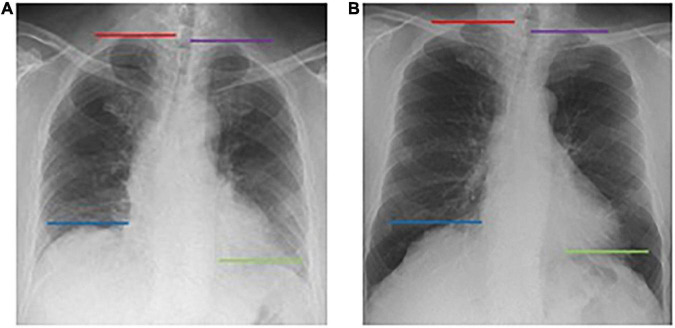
The Kinetic Analysis Tool™ automatically recognizes the bilateral pulmonary apexes and diaphragm dome. Dynamic X-ray working station automatically marked the bilateral diaphragm dome (right diaphragm: blue line; left diaphragm: green line) and lung apex (right lung: red line; left lung: purple line) in both end exhalation (A) and end inhalation (B), and tracing the position change with breathing.
